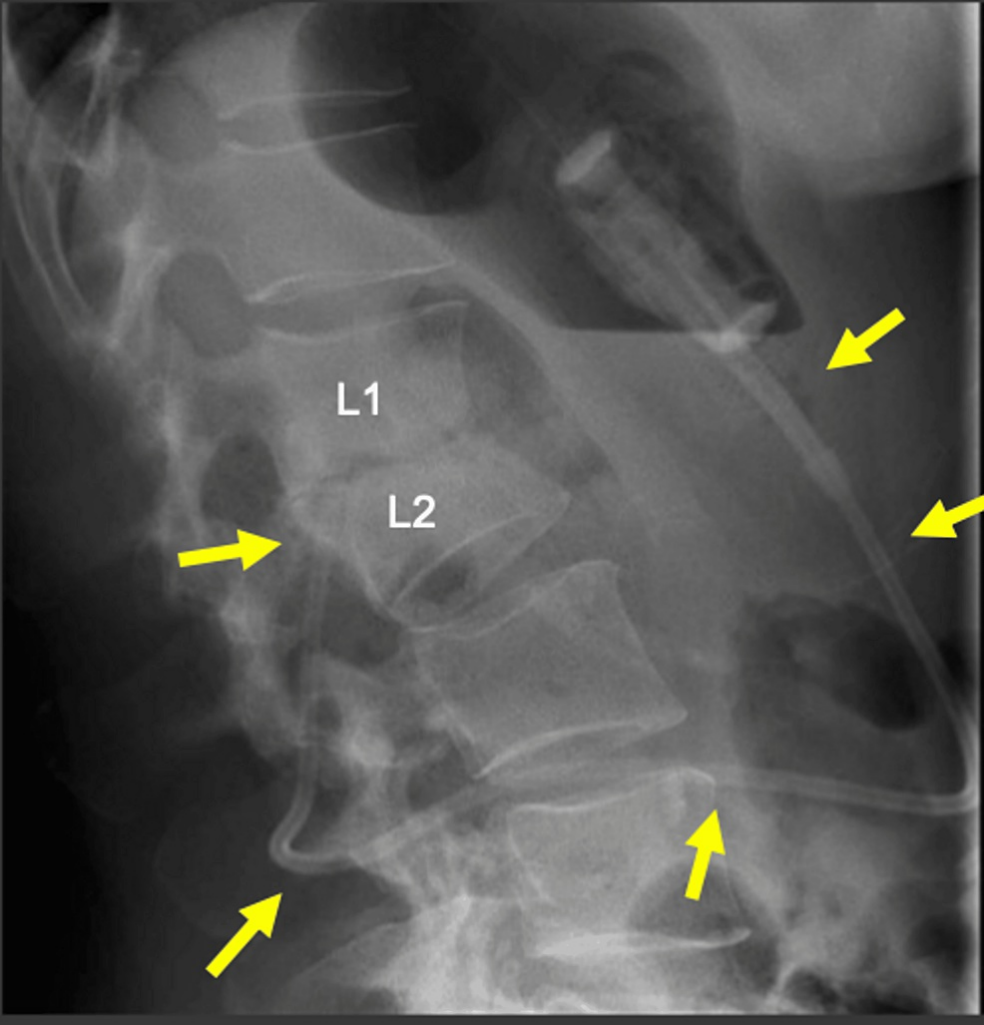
Lumbar spine x-ray showed height loss of the L1-2 intervertebral disc and erosive changes of the adjacent endplates. A catheter fragment is also seen extending from the gastric band to the disc space.
